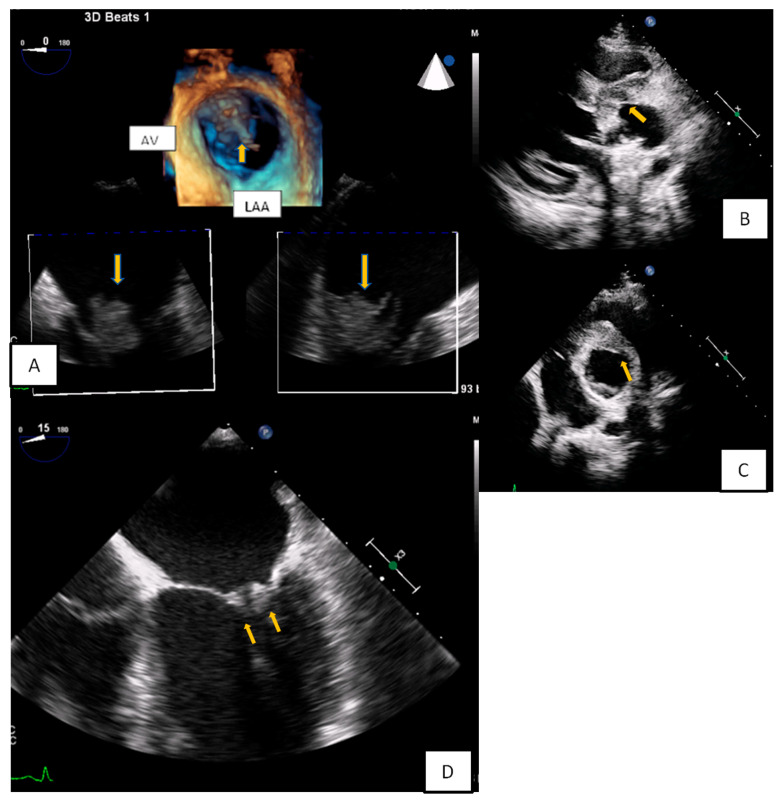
(A) 3D-TEE in a patient evaluated for a possible endocarditis. A large multilobular amorphous mass (vegetation) is found attached on the right commissure (3rd hour) of the anterior and posterior leaflet (AV: Aortic valve, LAA: Left atrial appendage). (B,C) A huge abscess infiltrating the aortic root and the aortomitral curtain in a young febrile patient with prosthetic aortic valve. (D) Transesophageal echocardiography-4 Chamber view. Increased thickness and echogenicity of both the tips of the leaflets in a patient with lupus erythromatosus endocarditis.
